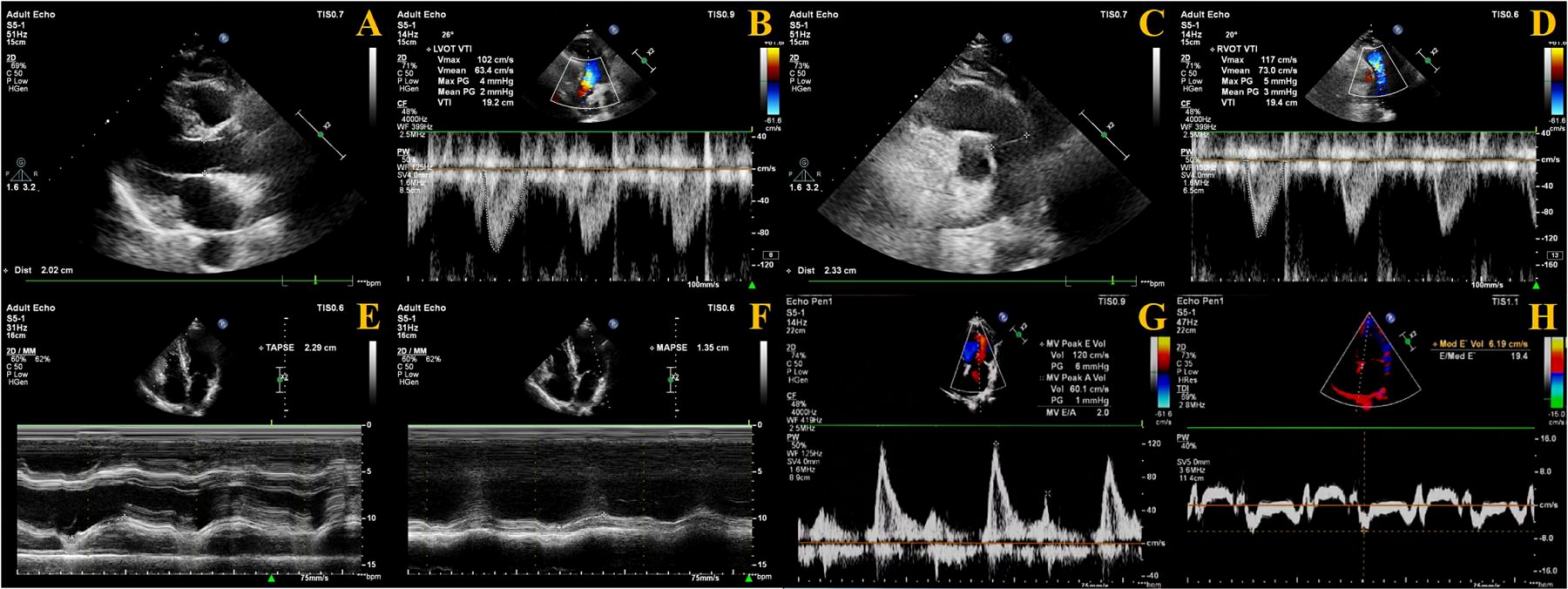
Measurement methods of echocardiography. (A) LVOT diameter measurement; (B) LVOT-VTI measurement; (C) ROVT diameter measurement; (D) RVOT-VTI measurement; (E) TAPSE measurement; (F) MAPSE measurement; (G) E velocity measurement; (H) Septal e′ measurement.
